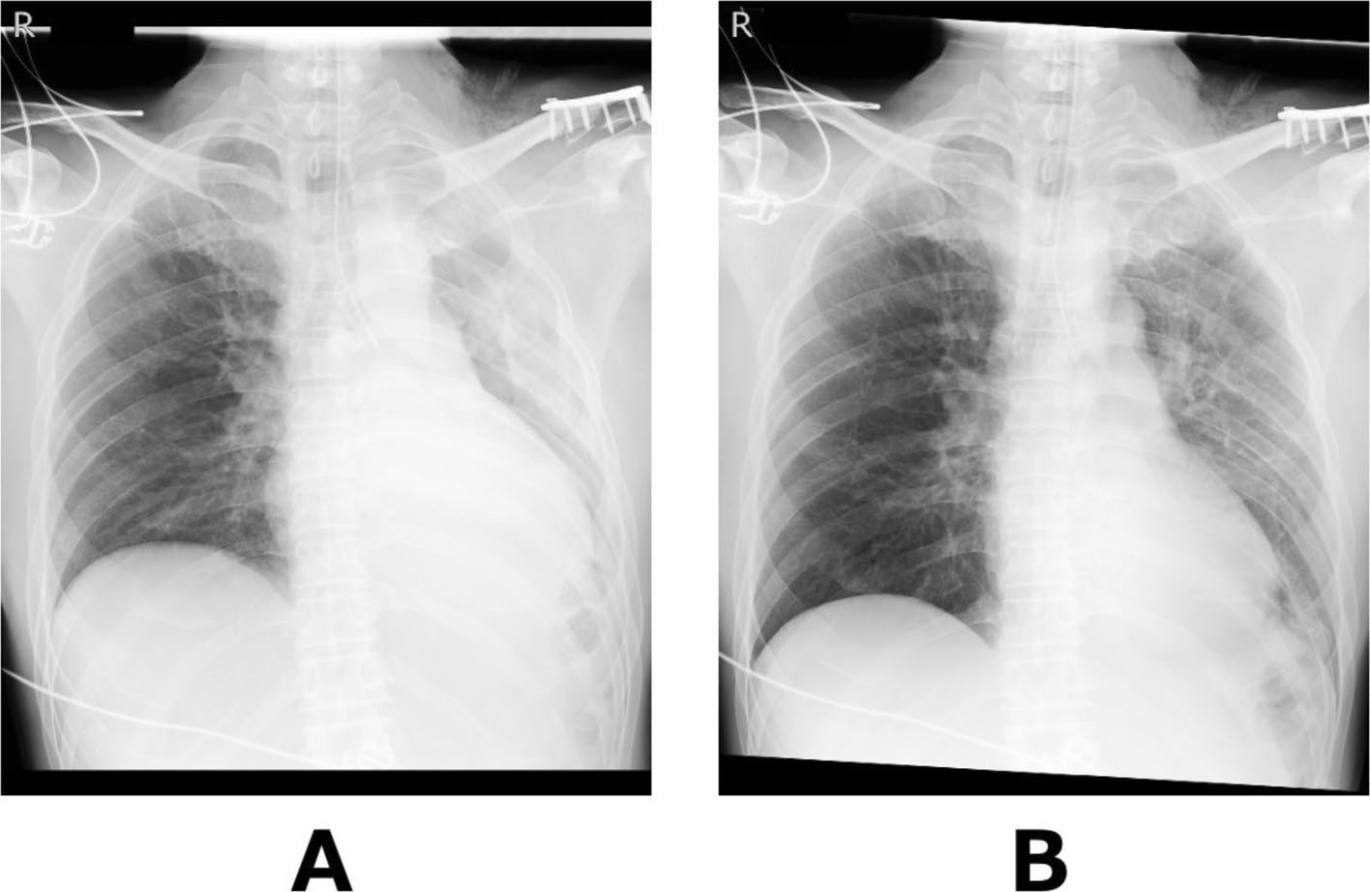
A Chest radiography while OLV was still in place showed decreased inflation of the left lung. B Left lung inflation improved after about one minute of gentle manual ventilation
